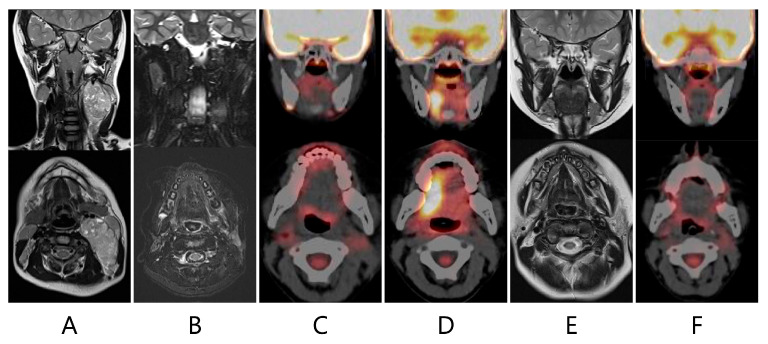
T2-weighted magnetic resonance imaging (MRI) showed a mass with heterogeneous signal intensity (54 × 48 × 33 mm) compressing the left carotid artery and internal jugular vein (A). MRI and 18F-FDG positron emission tomography combined with computed tomography (PET-CT) performed 9 (B) and 12 (C) months after completion of the chemotherapy found no recurrence. Sixteen months after completion of the chemotherapy, PET-CT revealed a novel area of glucose hypermetabolism in the right side of the tongue (SUV_max_ 10.79) (D). MRI performed at the same time demonstrated no evidence of a recurrence (E). Twenty months after completion of the chemotherapy, the FDG uptake in the tongue was no longer visible on PET-CT (F).
